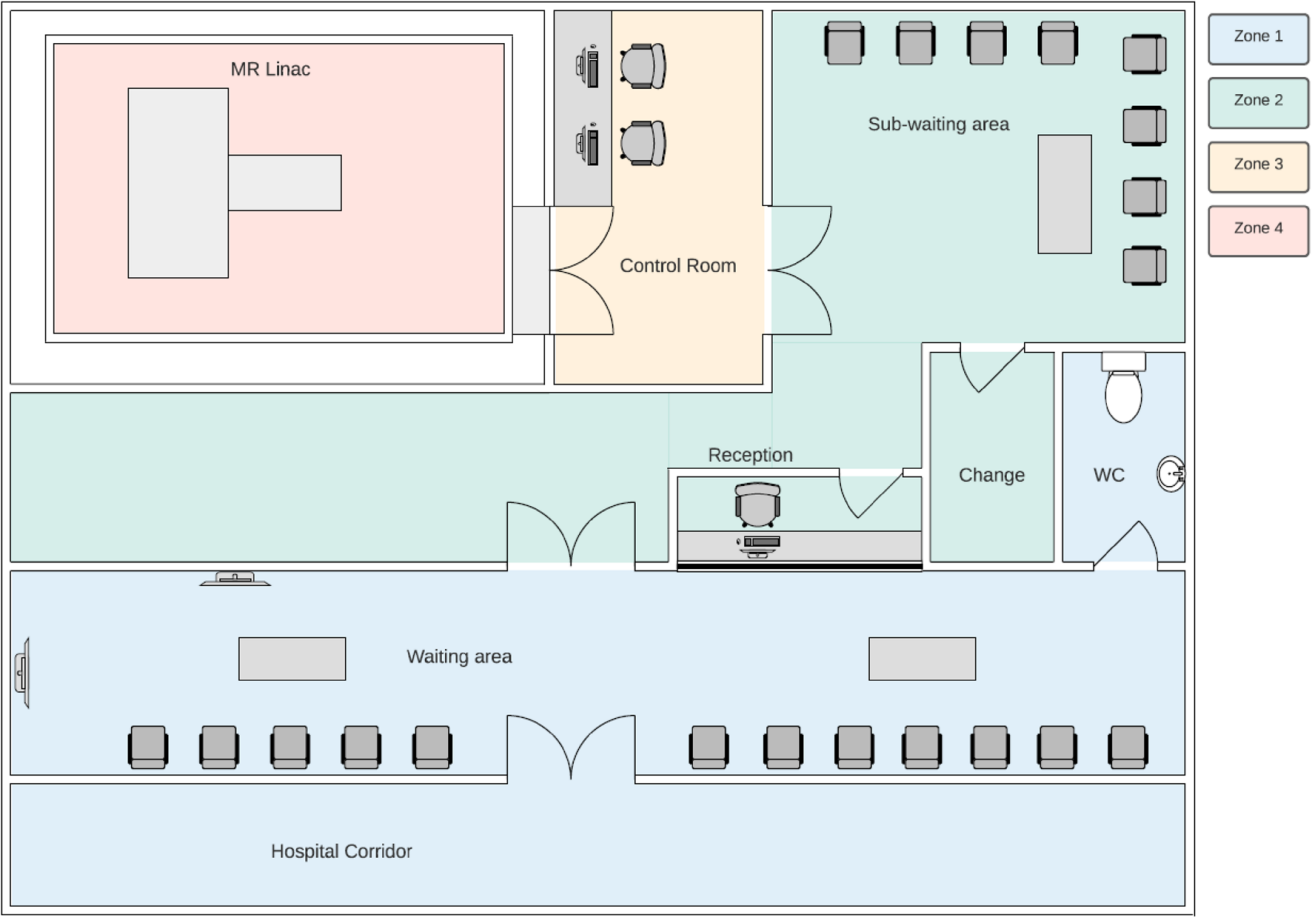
Example layout of MRI-linac suite showing demarcated safety zones
